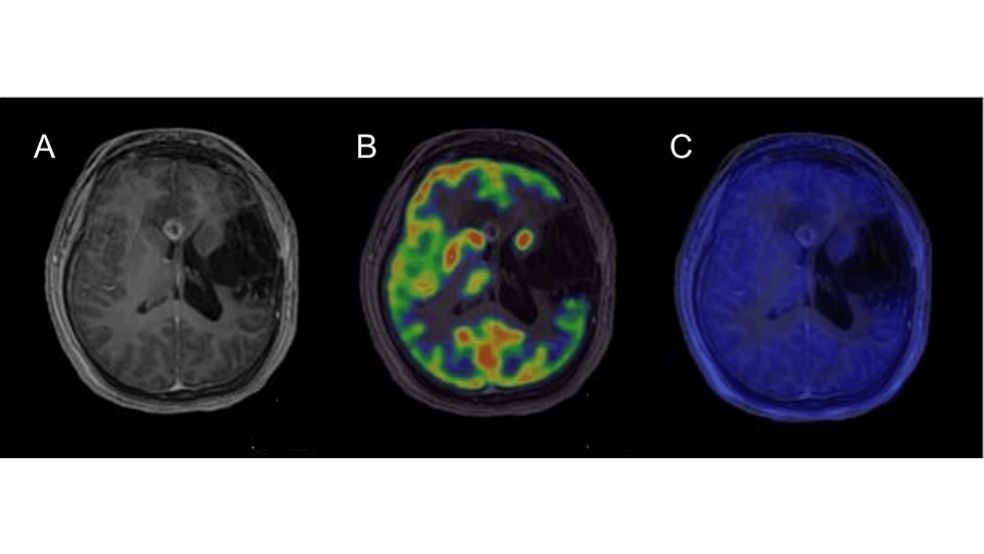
PET findings confirmed the presence of radionecrosis. (A) Located at the corpus callosum knee and was not associated with metabolism. (B) Positron emission tomography-fluorodeoxyglucose (PET-FDG) imaging with postsurgical changes at the level of the corpus callosum showed an ill-defined hyperdense image in its genual portion not associated with metabolism. Fused PET/MRI demonstrated the lesion in the previous MRI. (C) PET-acetate imaging with MRI fusion showed that the lesion did not present an increase in the transport and synthesis of fatty acids.
